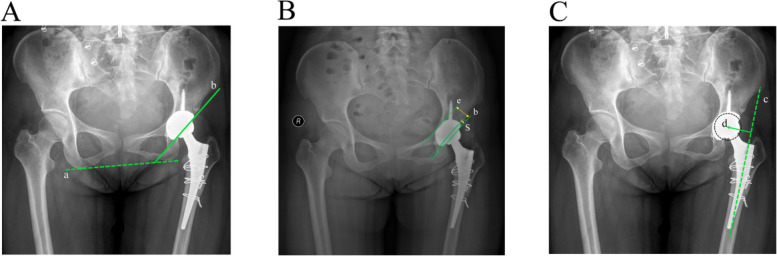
Measurement of prosthesis position on radiographs. Reference points and lines: (a) line through the ischial tuberosities; (b) line connecting the inner and outer edges of the acetabular cup; (c) longitudinal axis of the femoral stem; (d) centre of the prosthetic femoral head; (e) medial apex of the acetabular cup. A Acetabular inclination angle (AIA) is defined as the angle between line (b) and line (a). B Acetabular anteversion angle (AVA), where S is the transverse diameter of the cup ellipse, and eb is the distance from the outer rim of the acetabular cup to the medial apex; AVA was calculated as: AVA = 48.05 × (S/eb) − 0.3. C Femoral offset (FO) is defined as the perpendicular distance from point (d) to line (c)
